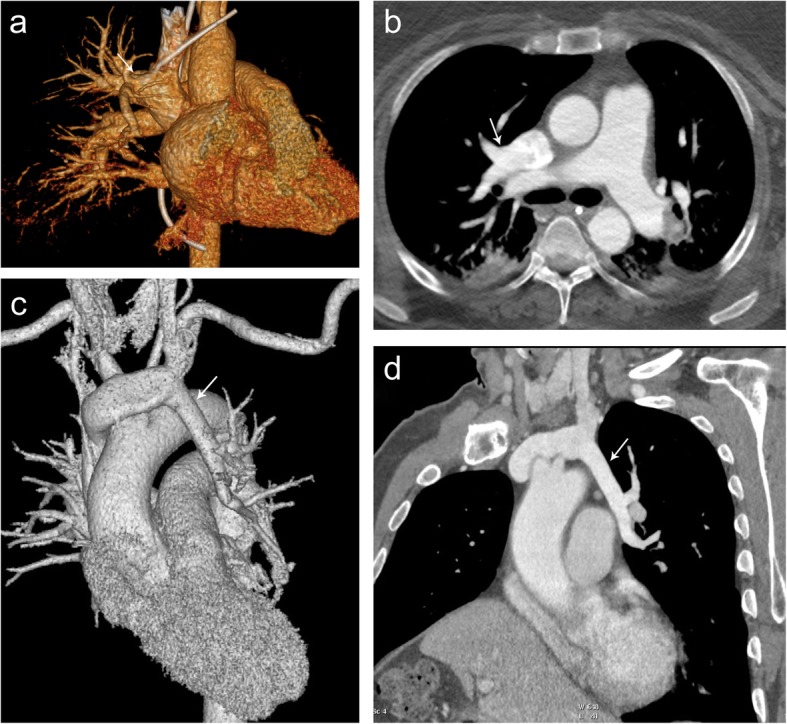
Internal jugular vein thrombus. Coronal contrast CT image demonstrating a catheter within the right internal jugular vein and nonopacification of the vessel, findings consistent with right internal jugular vein catheter-associated thrombus
